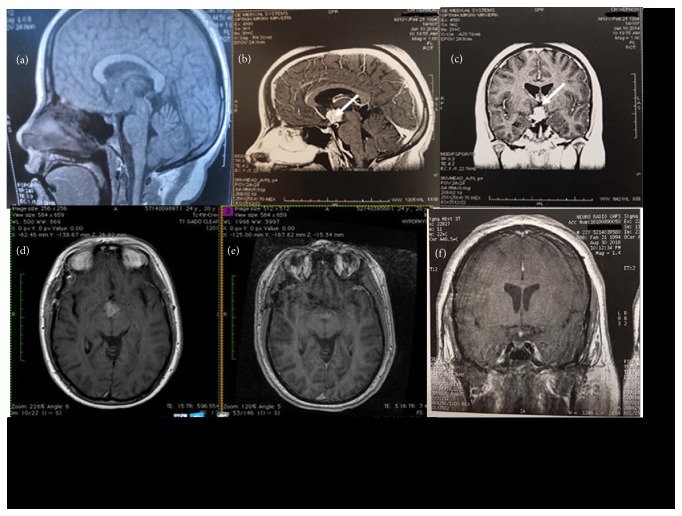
Evolution of brain MRI during follow-up.* At diagnosis, the normal T1-weighted hyperintense signal of the posterior pituitary has disappeared (a). Contrast-enhanced hypothalamic mass after gadolinium infusion ((b) and (c)); compression of third ventricle and thickening of pituitary stalk (b). Comparison of brain MRI (T1 with gadolinium) at diagnosis (M0) (d) and at the end of the first-line therapy (e). MRI showed a significant decrease of the hypothalamic mass but the persistence of contrast enhancement after gadolinium. Brain MRI (T1 after gadolinium infusion) at M30 showing a hypothalamic lesion stable in size and with decreased hypersignal compared to previous MRI (f).
